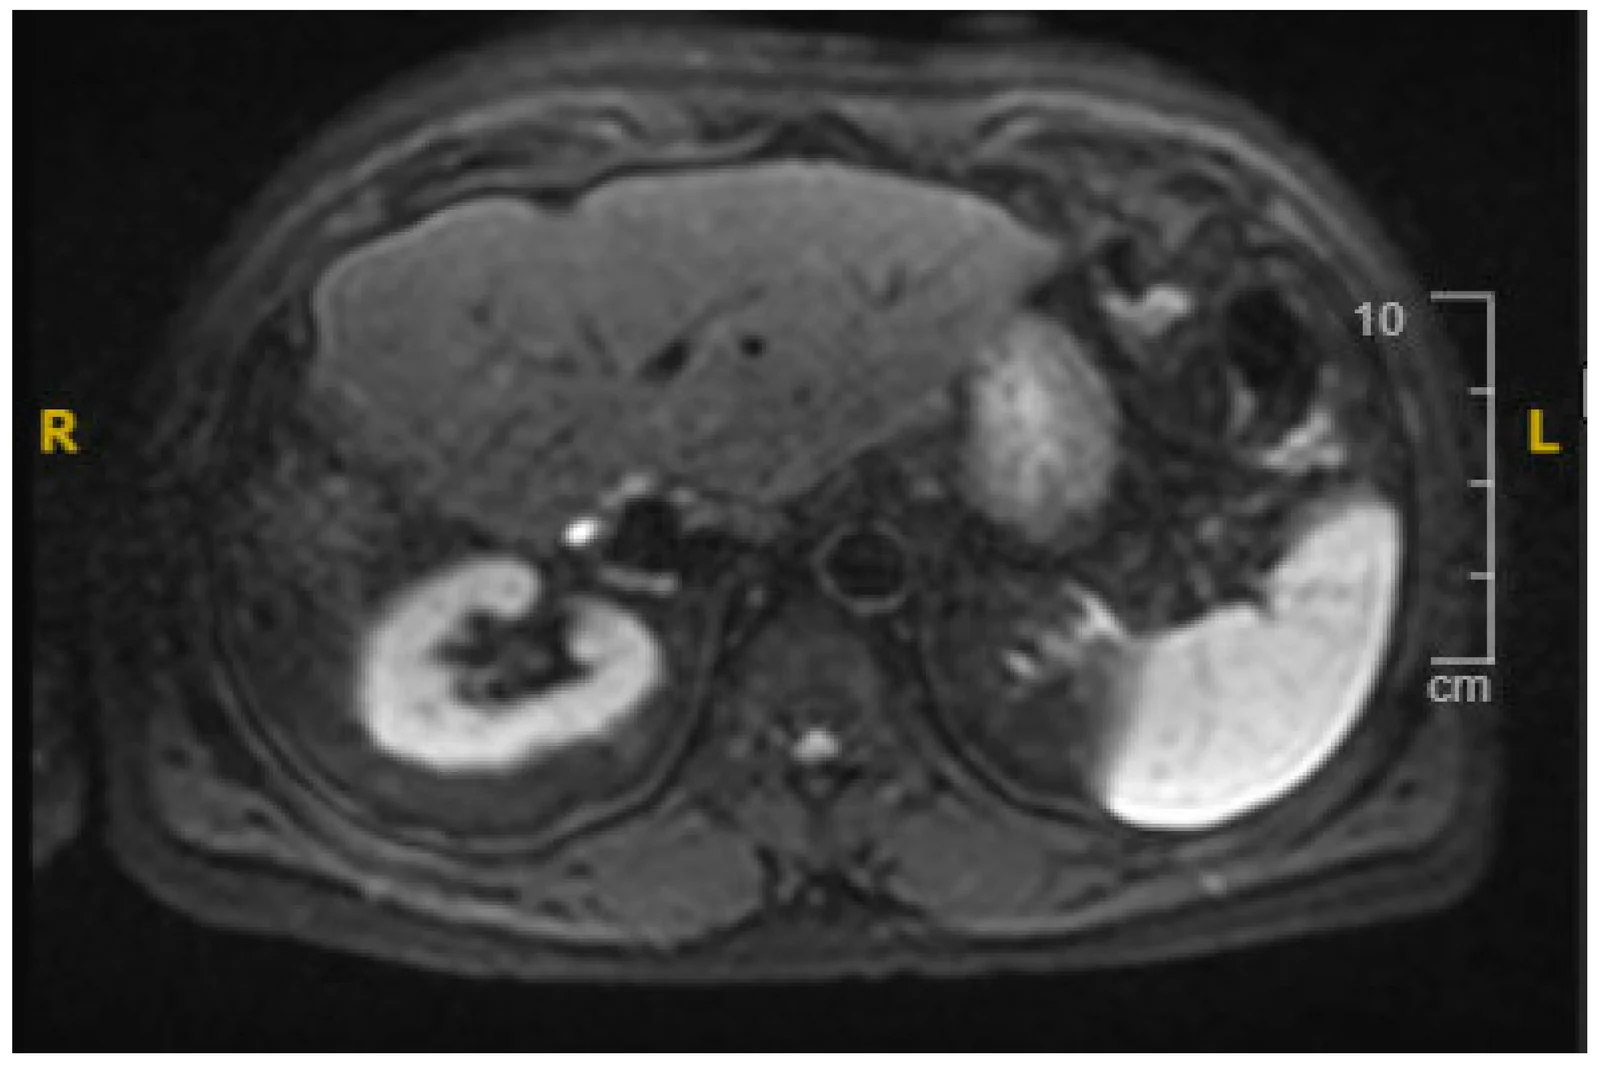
Post-hepatectomy follow-up MRI demonstrating stable postoperative hepatic changes without evidence of active intrahepatic recurrence at that time.
